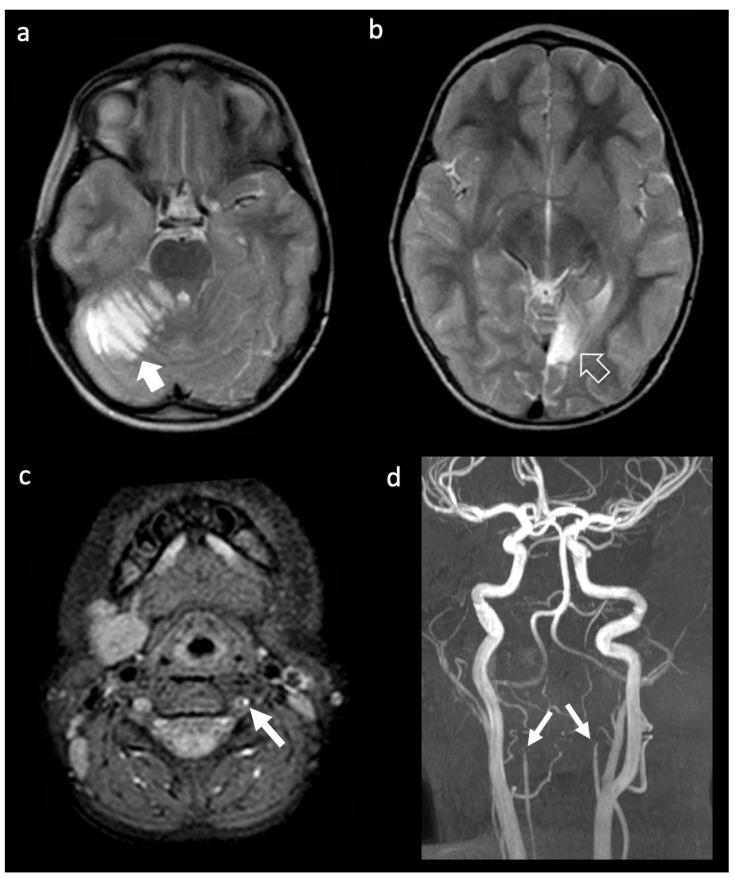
Neuroimaging findings of a pediatric patient with ffPXE (Patient 1) with a multiple AIS caused by dissection of both vertebral arteries. (a,b) Axial T2-weighted images reveal an acute right cerebellar infarct on the right (thick arrow) and a chronic occipital infarction the left (empty arrow). (c) Axial fat-saturated T1-weighted image demonstrates an acute hematoma within the wall of the left vertebral artery (thin arrow). (d) MRA, frontal view, shows bilateral interruption of the blood flow at V2-V3 level along the vertebral arteries (small thin arrows).
